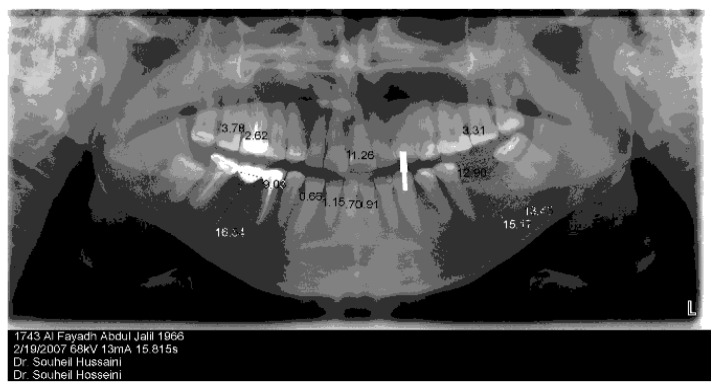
A panoramic radiograph demonstrating a radio-opaque marker.
